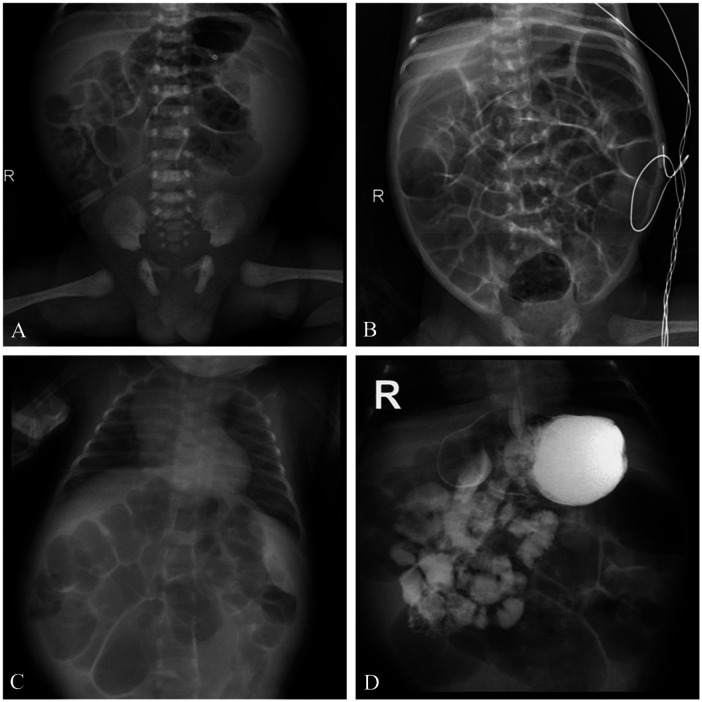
Abdominal x-ray; (A) Day 1 of life, (B) Day 7 of life, (C) Day 14 of life, (D) oral small bowel radiography. The abdominal x-ray on days 1, 7, and 14 of life revealed intestinal. Oral small bowel radiography at 70 days of life revealed a reversal of the jejunal and ileal locations, with right-sided jejunum and left-sided ileum.
